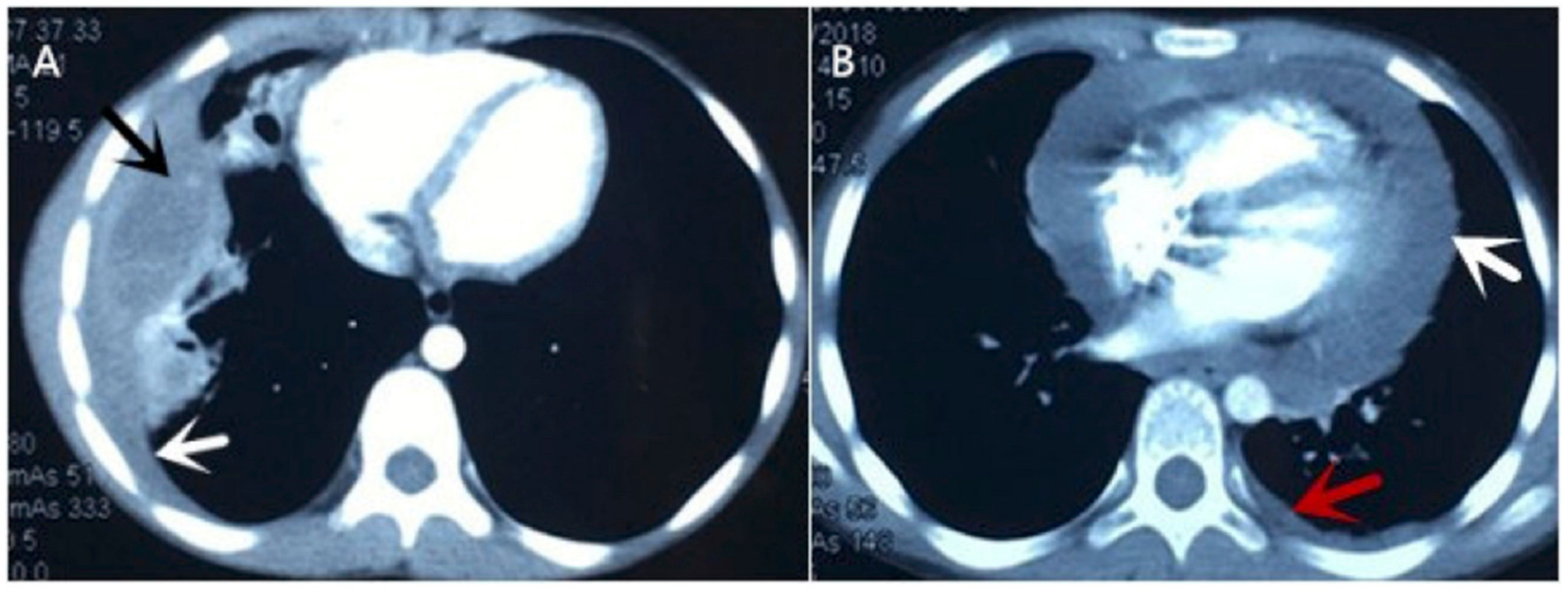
Chest CT of current cases. (A) It showed abscess in the middle and lower lobes of the right lung (black arrow), thickening of adjacent pleura, small amount of pleural effusion (white arrow). (B) It revealed large pericardial effusion (white arrow) and a small amount of pleural effusion on the left (red arrow).
